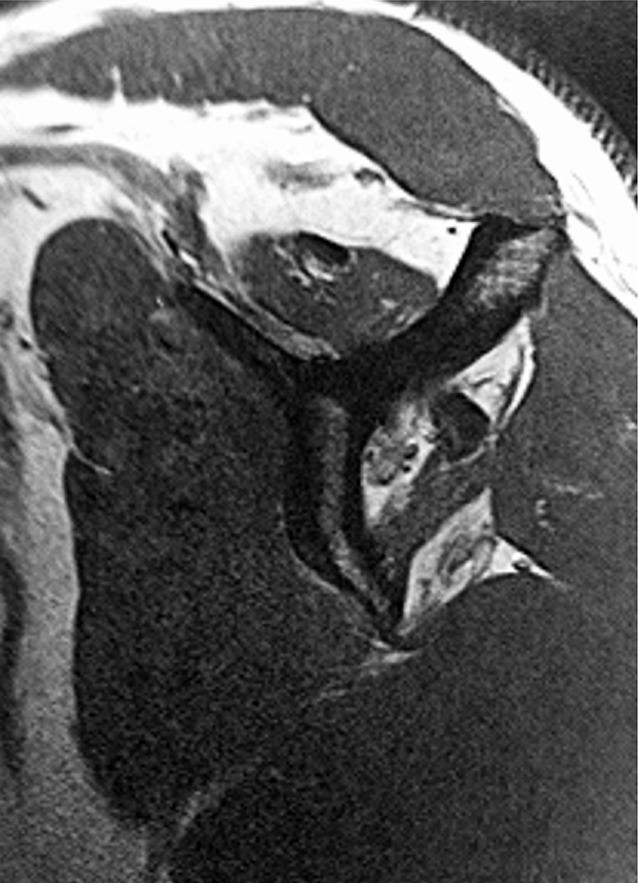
Magnetic resonance image showing atrophy of the supraspinatus, infraspinatus, and teres minor and an excellent subscapularis; clinically the patient has a lag sign and a drop sign and forward elevation is complete
